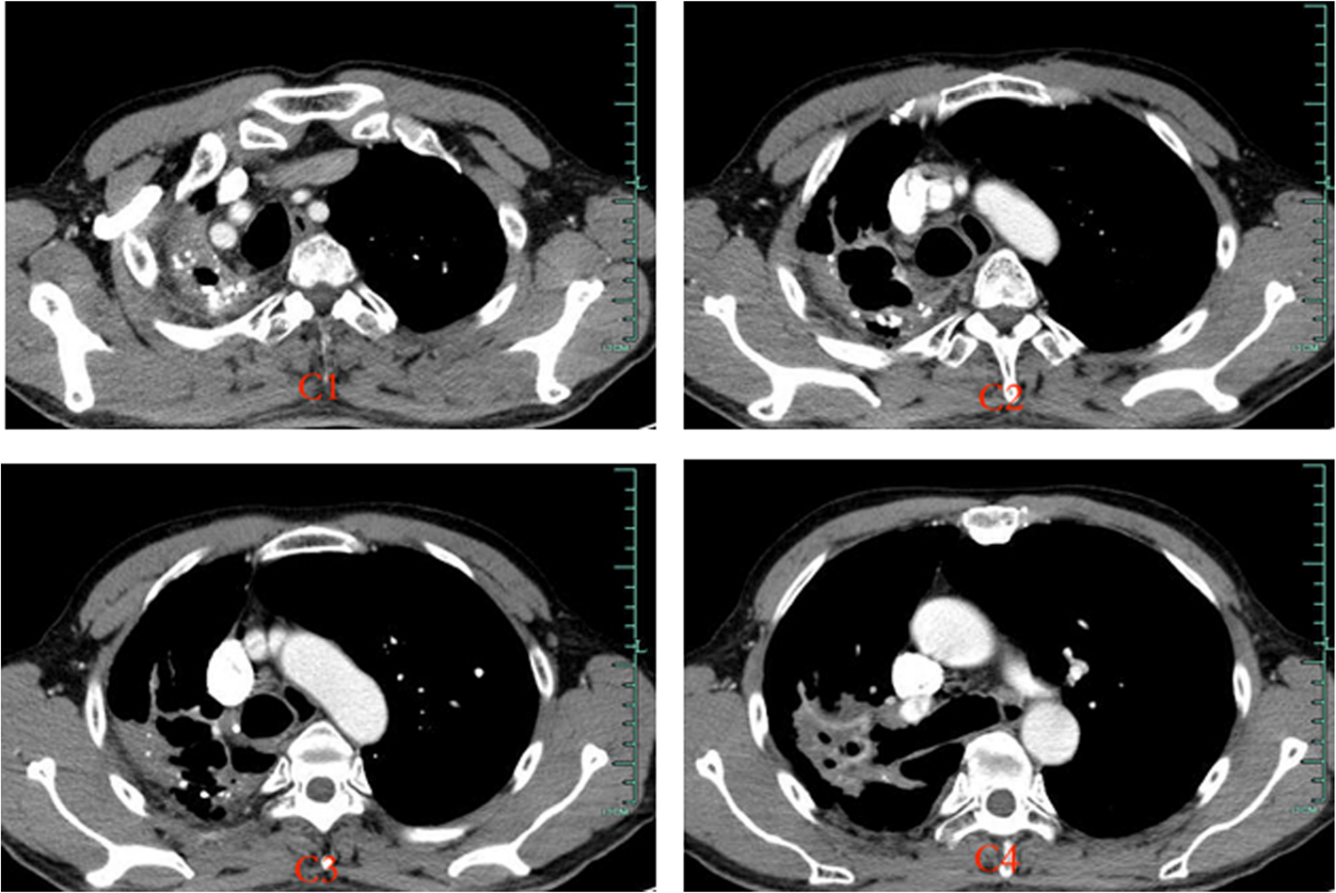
CT images taken 1 month after regional arterial embolization. (The same case as in Fig. 1):vascular enhanced CT showed a marked reduction in blood supply at the pleura, compared with the time before embolization
